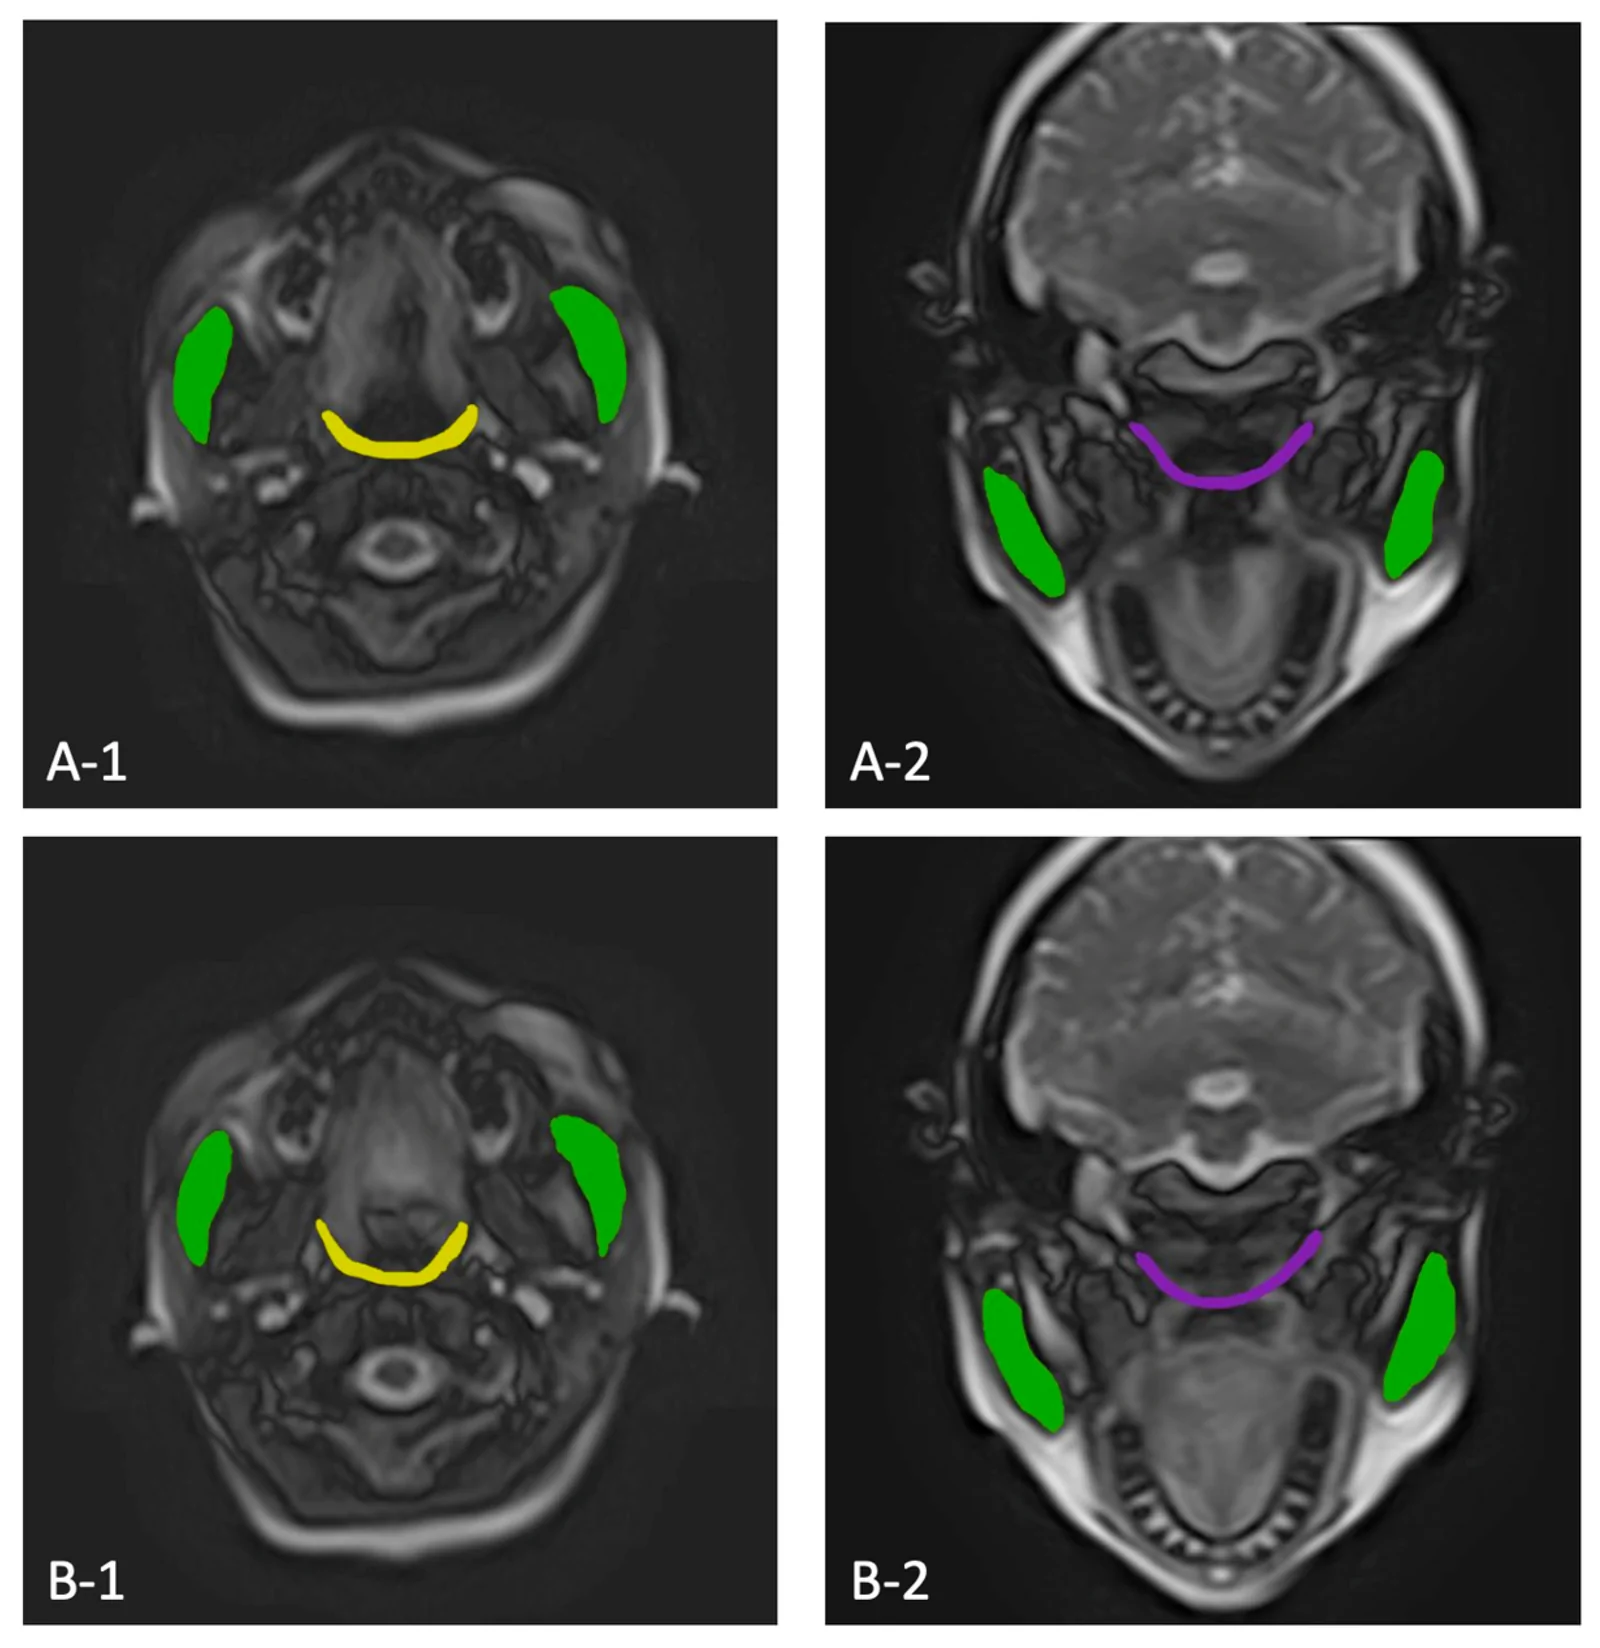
The signal intensities were calculated on cine-MRI during rest (A) and swallowing (B). The signal intensities of the LVPMs (purple), SPCMs (yellow), and MMs (green) were measured on axial images (A-1,B-1) and oblique coronal images (A-2,B-2).
